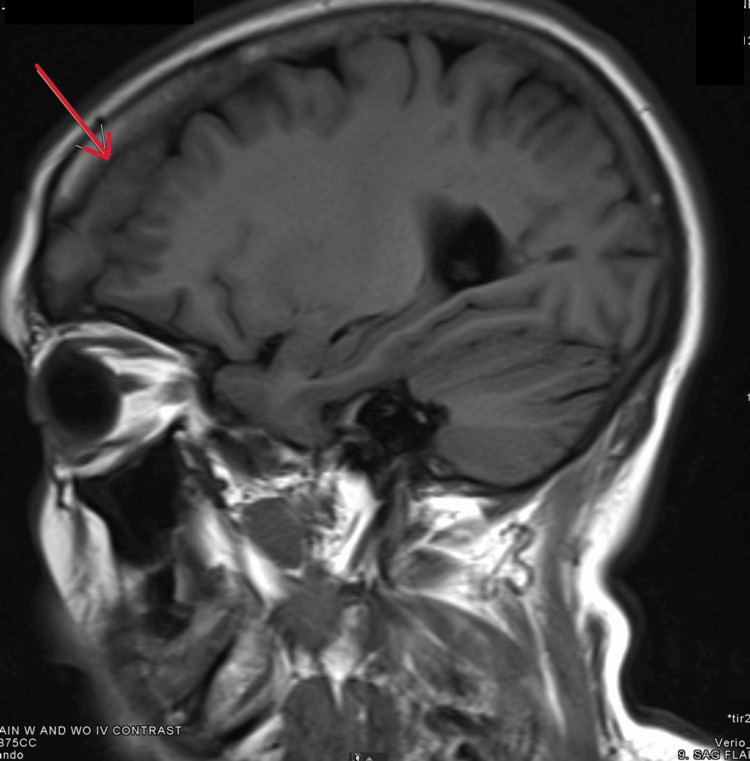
MR T1 FLAIR sequence, sagittal view, demonstrating isointense extradural intracranial extension of allergic fungal material along the anterior cranial fossa (red arrow). FLAIR, fluid-attenuated inversion recovery; MR, magnetic resonance
